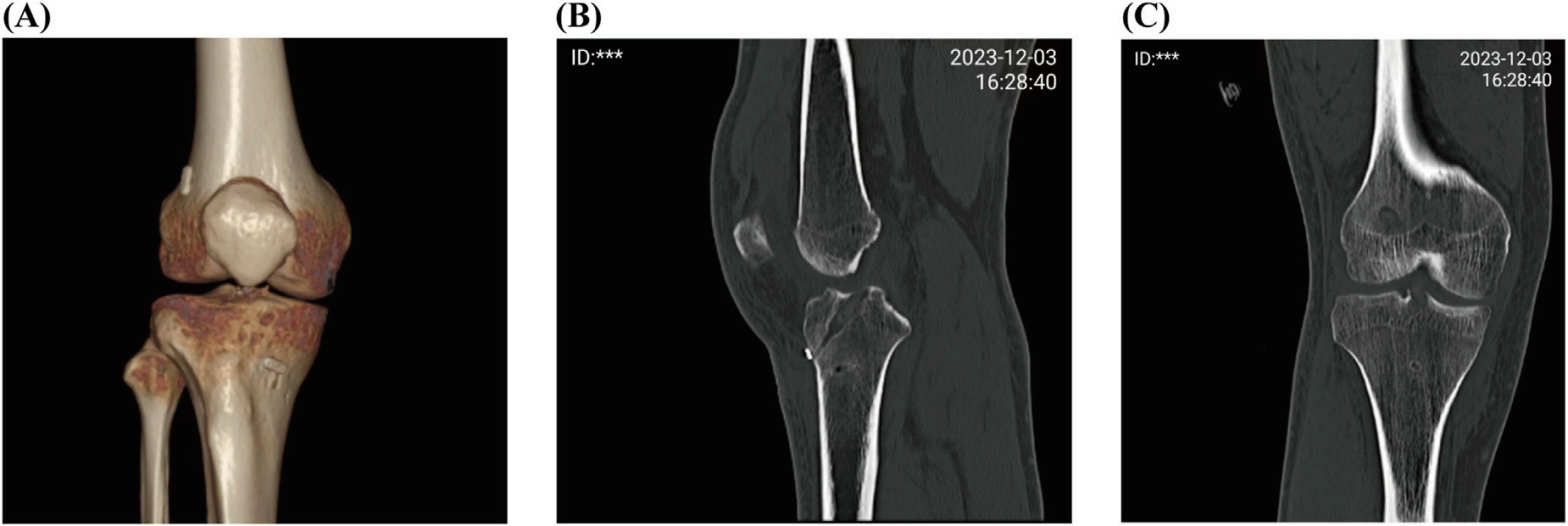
CT results. (A) is a three-dimensional reconstructed image. (B) is a sagittal CT image. (C) is a coronal CT image.
